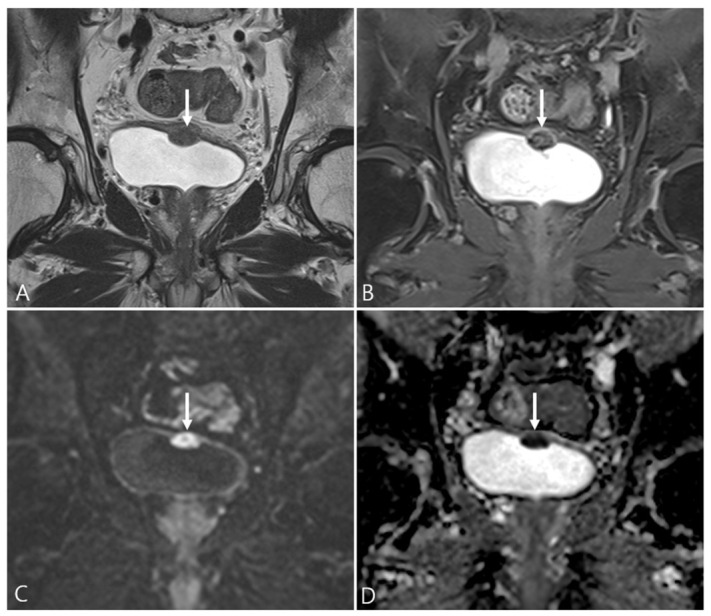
VI-RADS 4—Muscle-invasive bladder cancer at the bladder dome. An 83-year-old male presenting with a sessile mass at the bladder dome. Coronal images were preferentially acquired to optimize evaluation of the dome lesion, given its anatomical location at the superior aspect of the bladder. (A) Coronal T2-weighted imaging (T2WI) demonstrates an ovoid shaped mass at the bladder dome with disruption of the low-signal muscularis propria; the tumor stalk sign is not identified, raising concern for muscle invasion. (B) Coronal T1-weighted contrast-enhanced imaging (T1CE) shows early enhancement extending into the muscularis propria, supporting the likelihood of muscle-invasive disease. (C) High b-value diffusion-weighted imaging (DWI; b = 1000 s/mm^2^) demonstrates marked diffusion restriction within the tumor without the characteristic inchworm sign. (D) Apparent diffusion coefficient (ADC) map confirms significantly reduced ADC values, consistent with high tumor cellularity. The overall imaging features are consistent with a VI-RADS score of 4, indicating a high probability of muscle invasion. Subsequent TURBT confirmed pathological stage T2 urothelial carcinoma with concomitant carcinoma in situ (CIS). Given the patient’s refusal of radical cystectomy, concurrent chemoradiotherapy (CCRT) was administered as a bladder-preserving strategy, and the patient is currently under follow-up.
